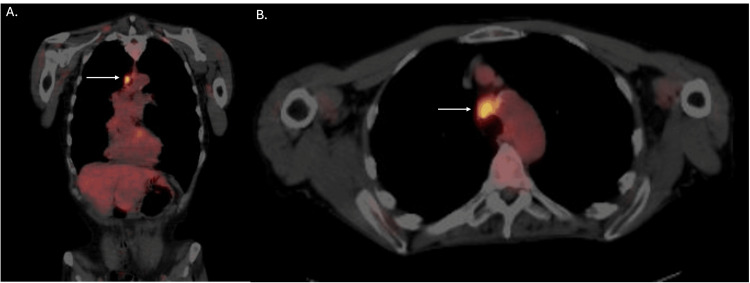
Pre-treatment FDG-18 PET/CT of the chest. (A) Coronal view. (B) Axial view. Imaging reveals a 3.25 x 2.5 cm hypermetabolic mass in the anterior trachea with an SUVmax of 7.65
